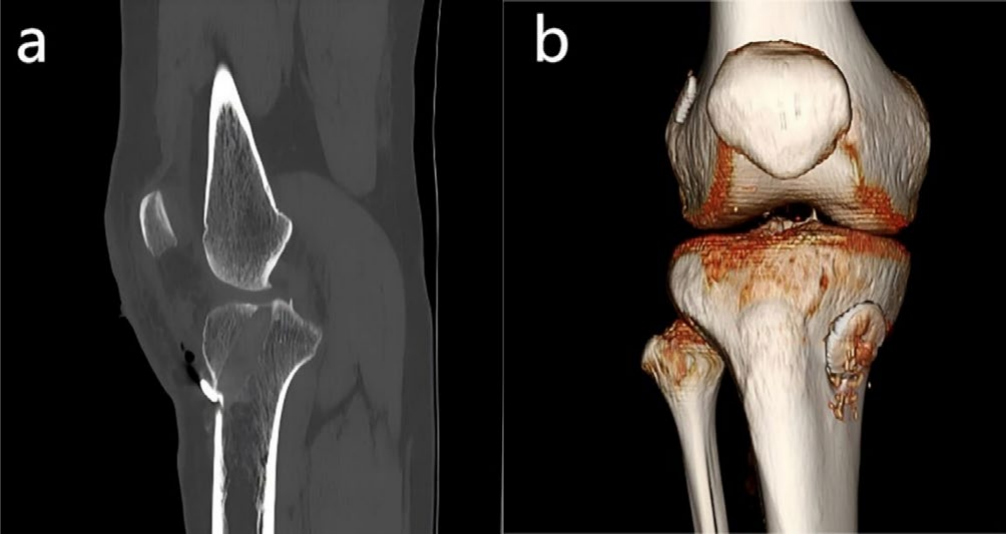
Postoperative computed tomography (CT) images demonstrating tibial tunnel position after single‐bundle anterior cruciate ligament reconstruction. (a) Three‐dimensional volume‐rendered CT reconstruction of the knee showing the tibial tunnel aperture on the anteromedial aspect of the proximal tibia. (b) Sagittal CT reconstruction demonstrating the intraosseous trajectory of the tibial tunnel from the anteromedial cortex to the native ACL footprint on the tibial plateau.
